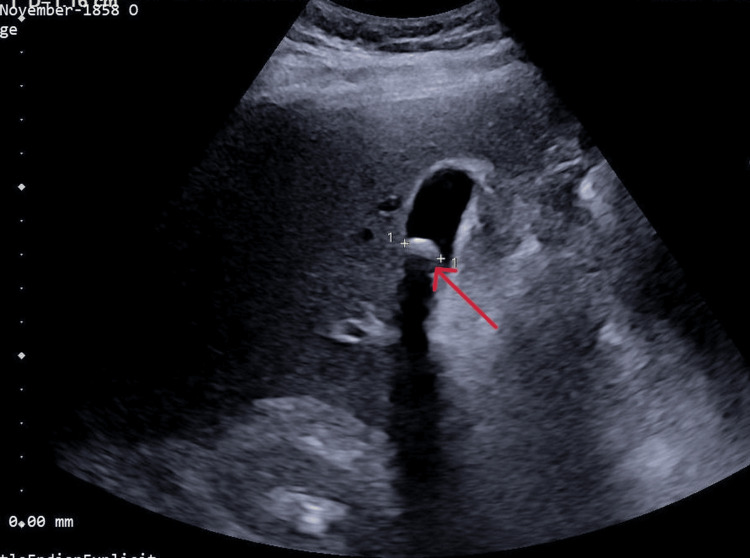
Ultrasonographic image of the patient The arrow indicates the gallbladder stone.
